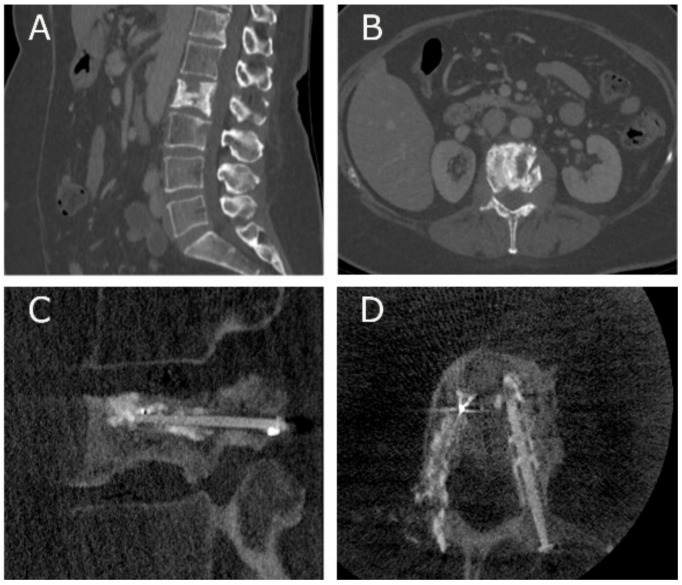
Pre-operative CT scans of the L2 metastatic lesion (A) sagittal, (B) transversal, and immediate post-operative fluoroscopic images of the PEEK (polyetheretherketone) implants (C) sagittal, (D) transversal.
